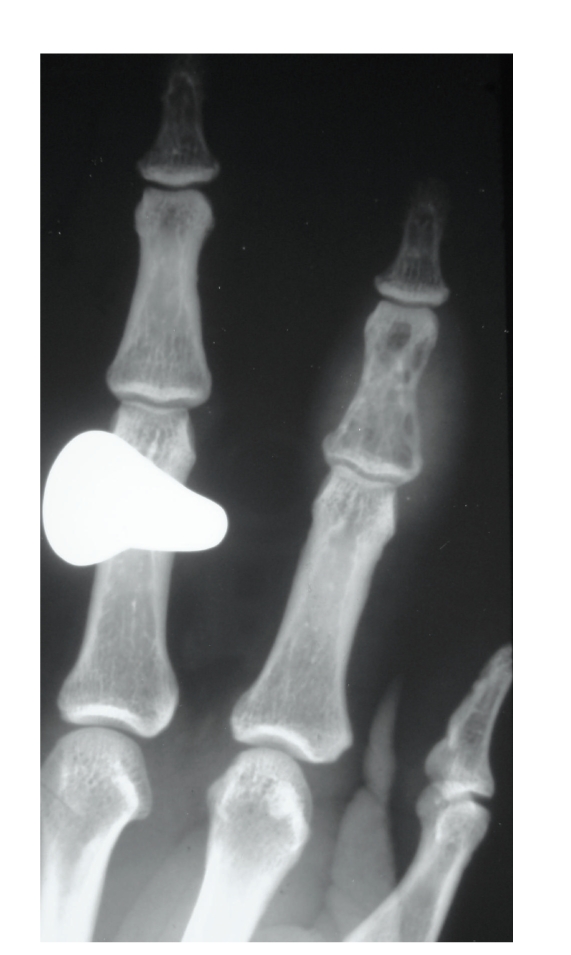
Plain radiograph of the hand of a patient with sickle cell anaemia showing patchy lucency and associated soft tissue swelling consistent with osteomyelitis of the middle phalanx of the index finger.
